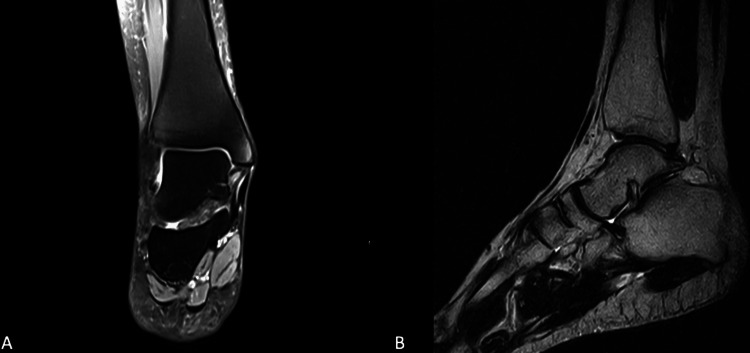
(A, B) MRI images for a 29-year-old male with chronic ankle pain and instability. Radiological diagnosis showed preservation of normal anatomy.
